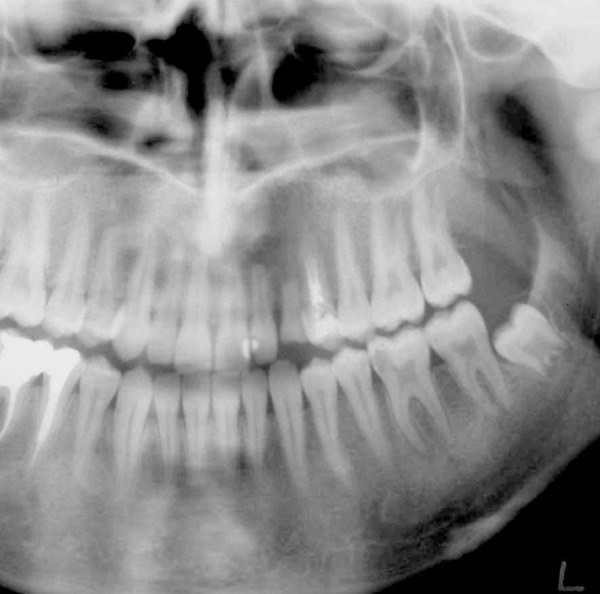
Orthopantomogram showing inadequate root canal filling of maxillary left first premolar
